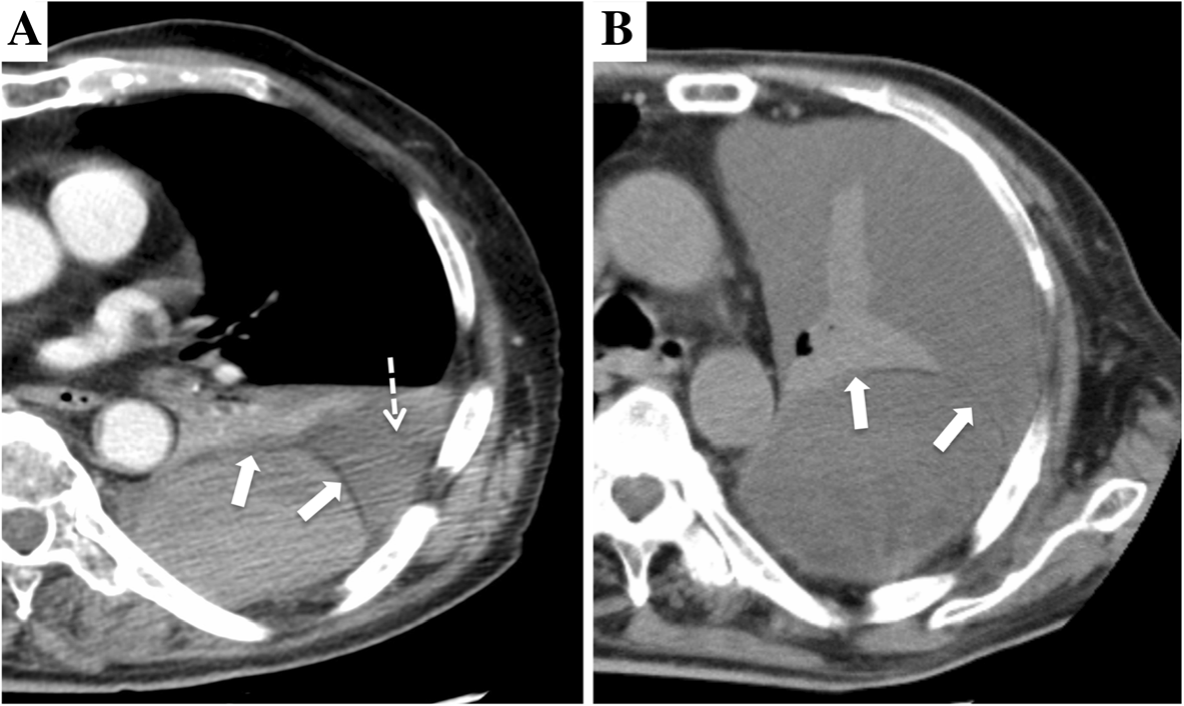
Chest computed tomography showing hemothorax (dashed arrow), a convex extrapleural hematoma with extrapleural fat sign (white arrow), and atelectasis of the left lower lobe on the patient’s posterior chest wall on admission (a) and a large amount of pleural effusion and atelectasis with a residual extrapleural hematoma (white arrow) 53 days after the episode of trauma (b)
